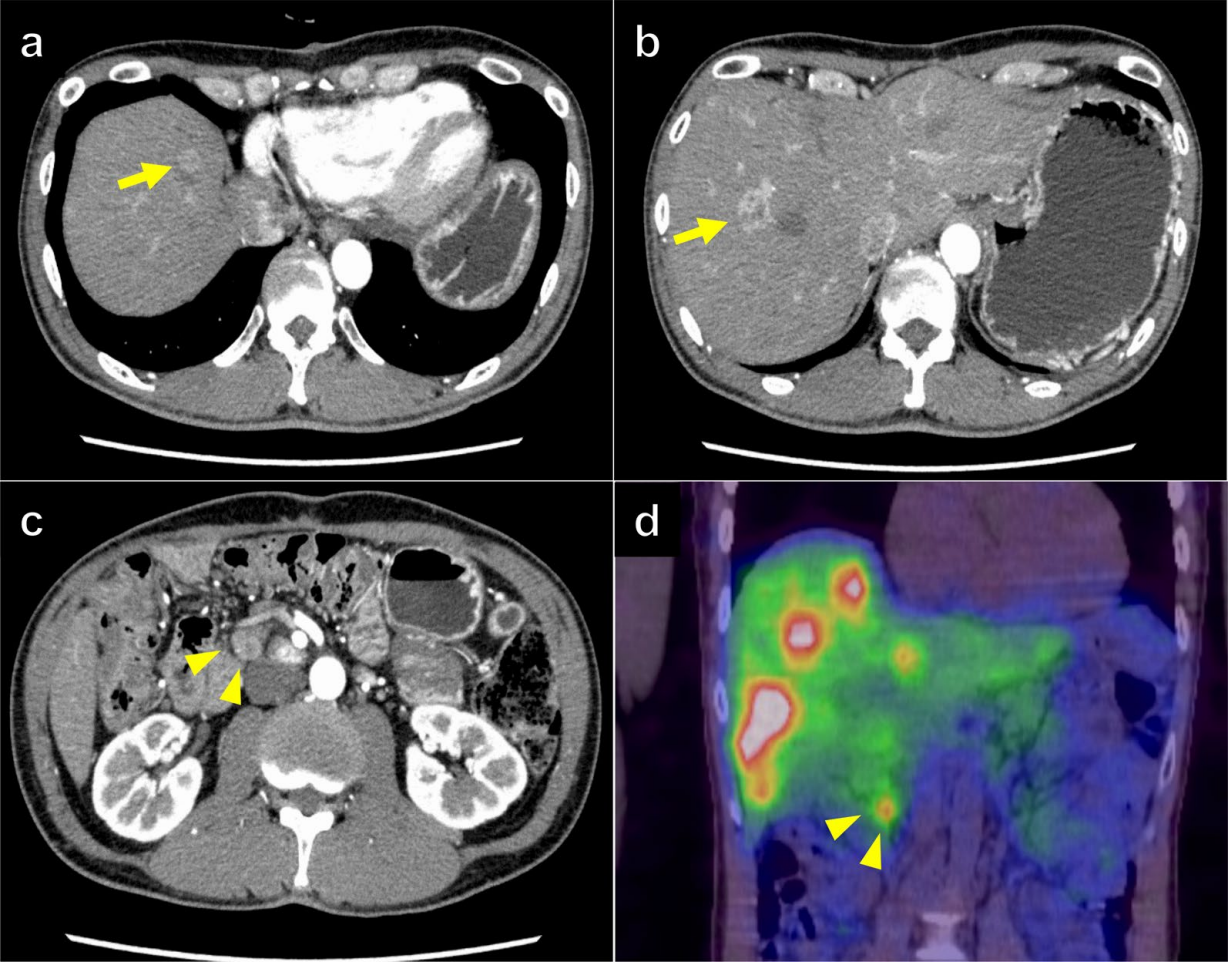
Second recurrence. Contrast-enhanced computed tomography revealed multiple liver metastases (arrows) (a and b) and lymph node metastasis near the superior mesenteric vein (arrowheads) (c). Somatostatin receptor scintigraphy revealed receptor accumulation in the liver and the lymph node (arrowheads) (d)
